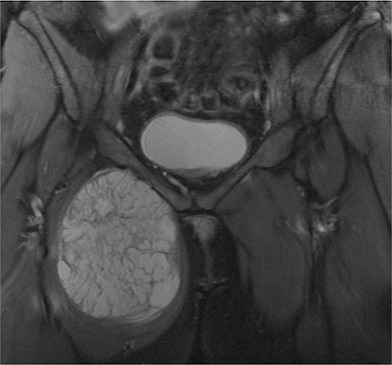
A 48-year-old woman had a Grade 2 peripheral chondrosarcoma of the right pubic bone. MRI shows a 13-cm-thick cartilage cap.
